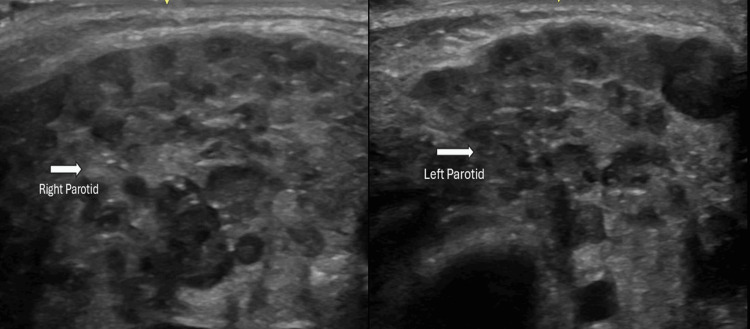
Bedside point of care ultrasound showing right and left parotid swelling
